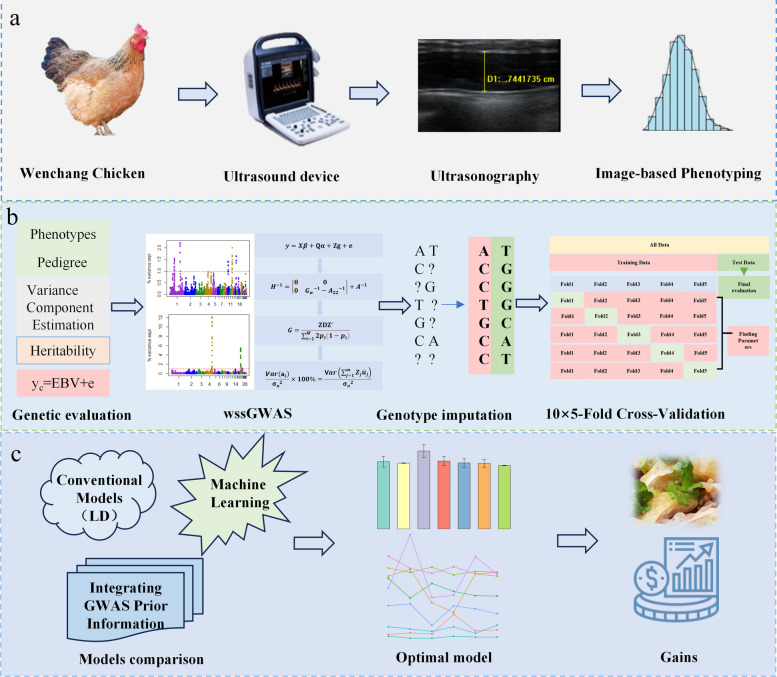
An integrated framework for precision breeding of chicken abdominal fat traits by combining ultrasound phenotyping and multi‑omics prediction
